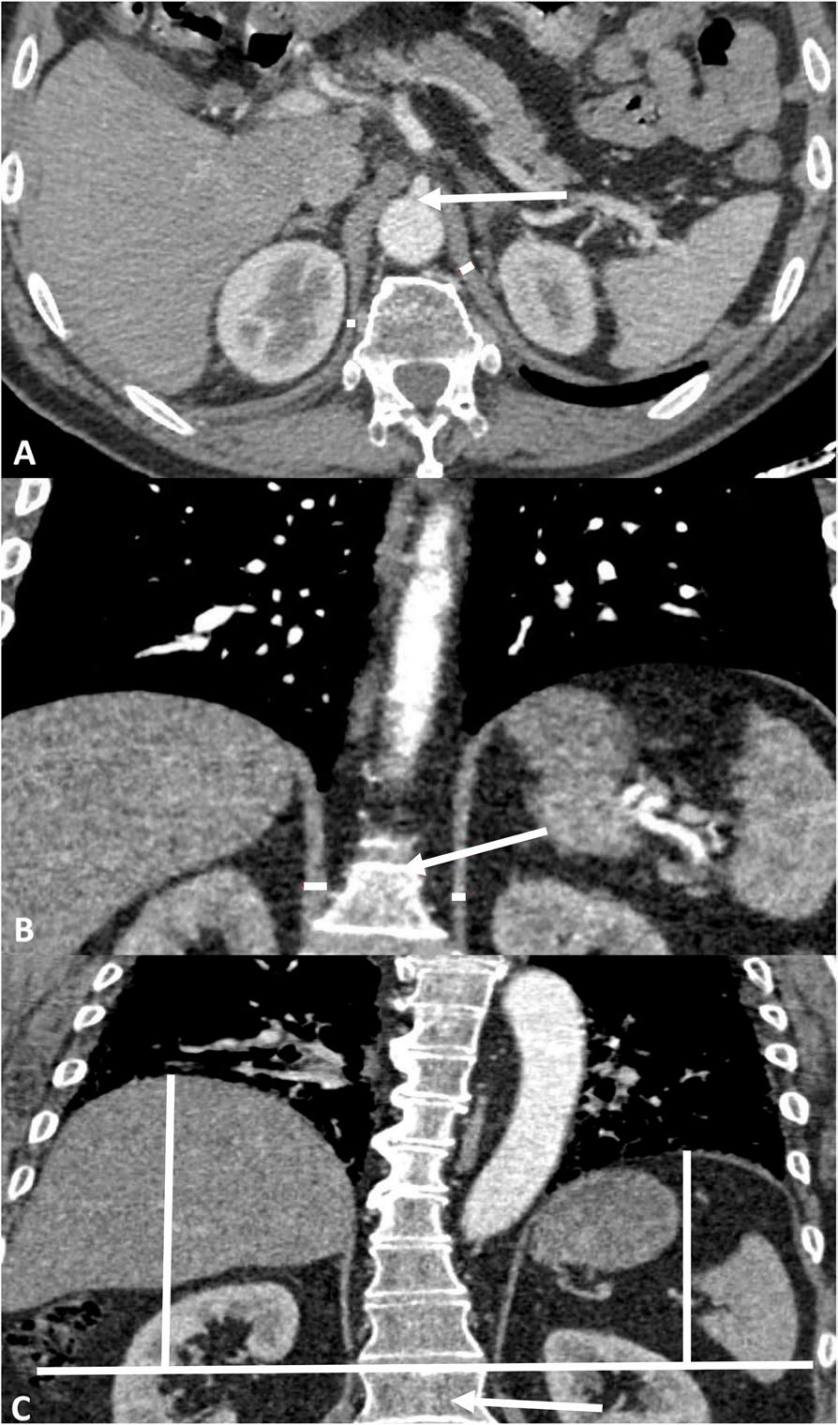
Measurements of the diaphragm performed on enhanced CT scan. A Measurement of the pillars at the level of the celiac artery. Minimal diaphragm thicknesses (red lines) at the level of the origin of the celiac artery (red arrow). B Measurement of the pillars at the level of L1. Diaphragm thicknesses (red lines) along the anterior part of the L1 vertebral body at mid-level (red arrow). C Measurement of the diaphragm height difference. Heights (red lines) from the highest point of the diaphragmatic dome to its perpendicular intersection with a line (white line) following the upper plateau of L1 (red arrow)
